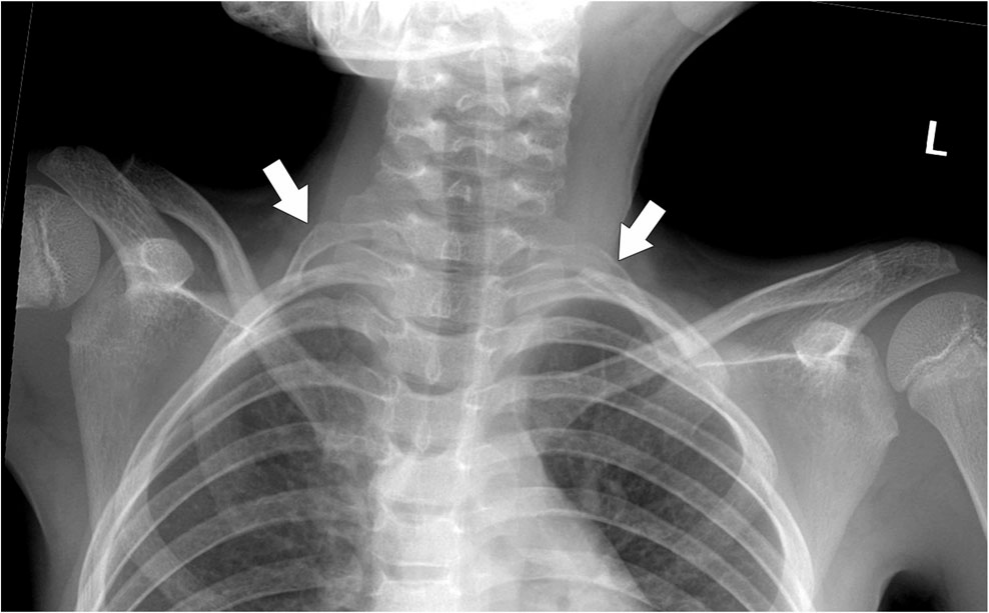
Chest X-ray demonstrating bilateral cervical ribs (indicated by the arrows)
